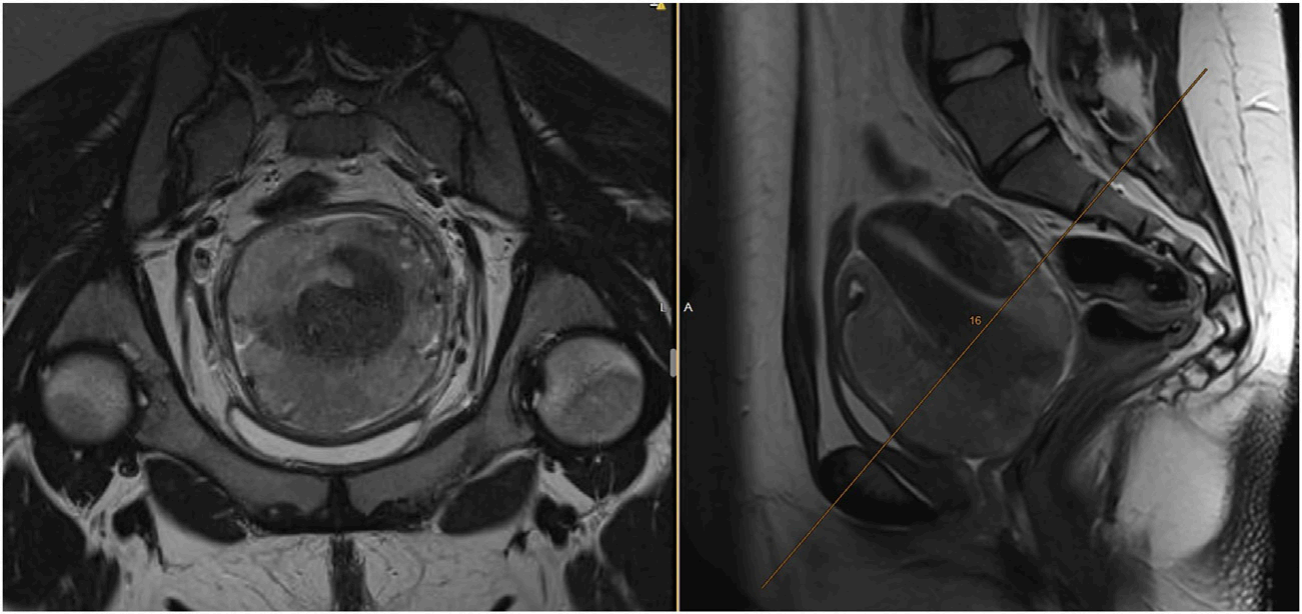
Baseline MRI of the uterine cervix SCCOHT. The cervical tumor grows atypically more around the uterus, than infiltrating it. Expansive tumor growth with pressing to the bladder or rectum, with no clear infiltration into adjacent organs.
